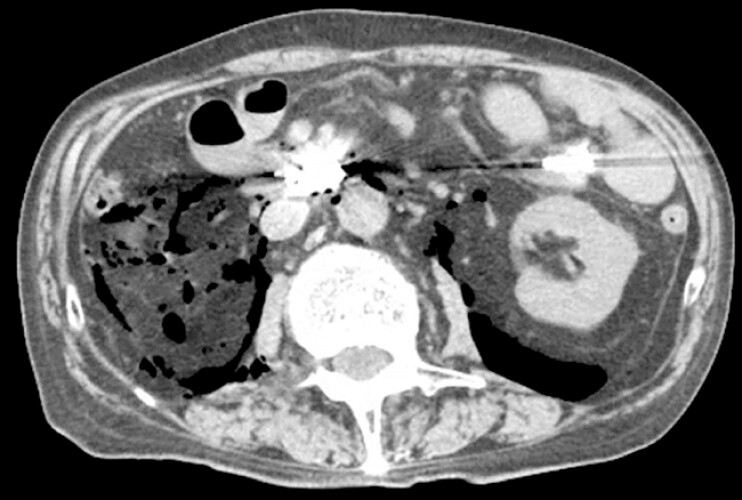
Postprocedural CT findings of mucosal closure and retroperitoneal free air. Postprocedural abdominal CT scan is performed. The CT image shows endoscopic clips securing the mucosal defect and free air in the retroperitoneal space.
